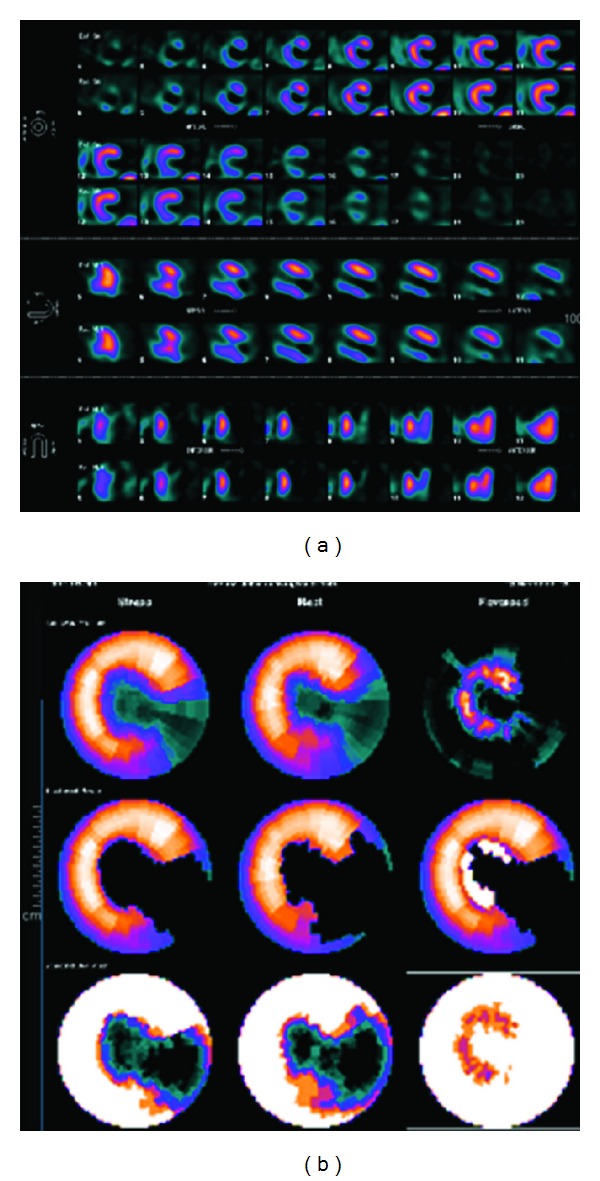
(a) The myocardial perfusion imaging with TC-99 M Sestamibi SPECT at rest and stress with dipyridamole demonstrated left ventricular lateral wall and apical transmural myocardial infarction without peri-infarction myocardial ischemia. (b) Bull's eye plots were created by automated quantitative analysis software, representing the stress and rest data.
